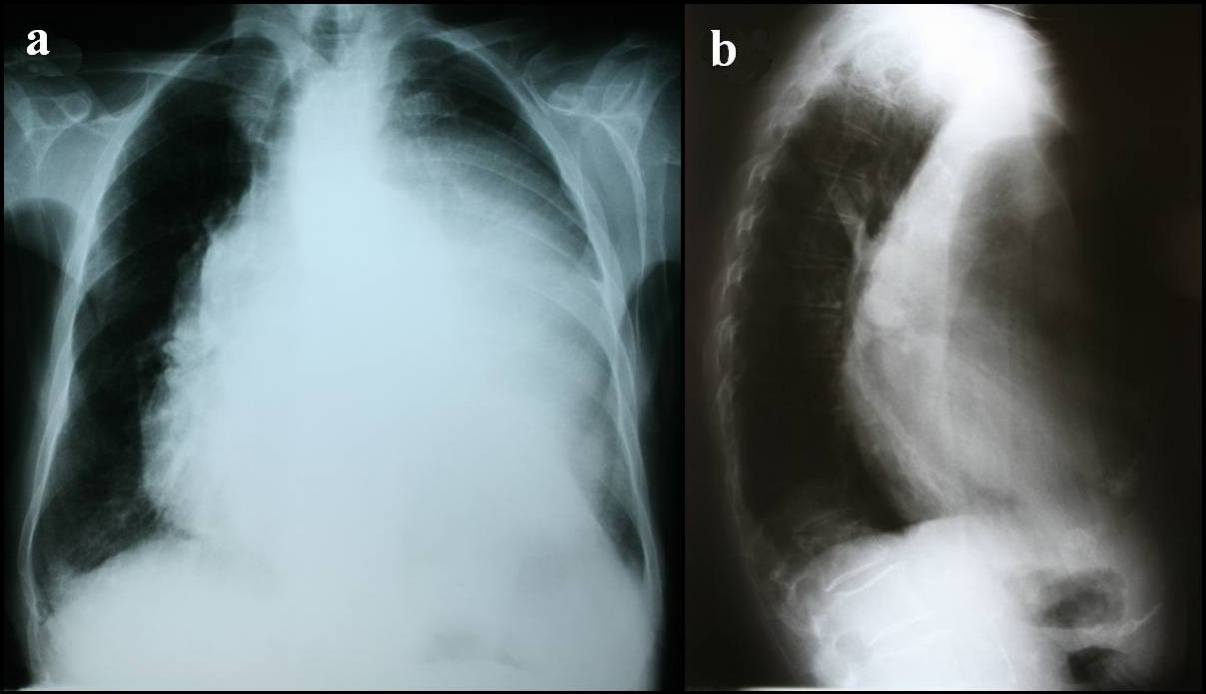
Pre-operative posteroanterior chest radiograph and lateral chest radiograph. Pre-operative (a) posteroanterior chest radiograph demonstrates a mediastinal mass that displaces the trachea and fills almost the entire left hemithorax. Lateral chest radiograph (b) shows mild kyphosis.
